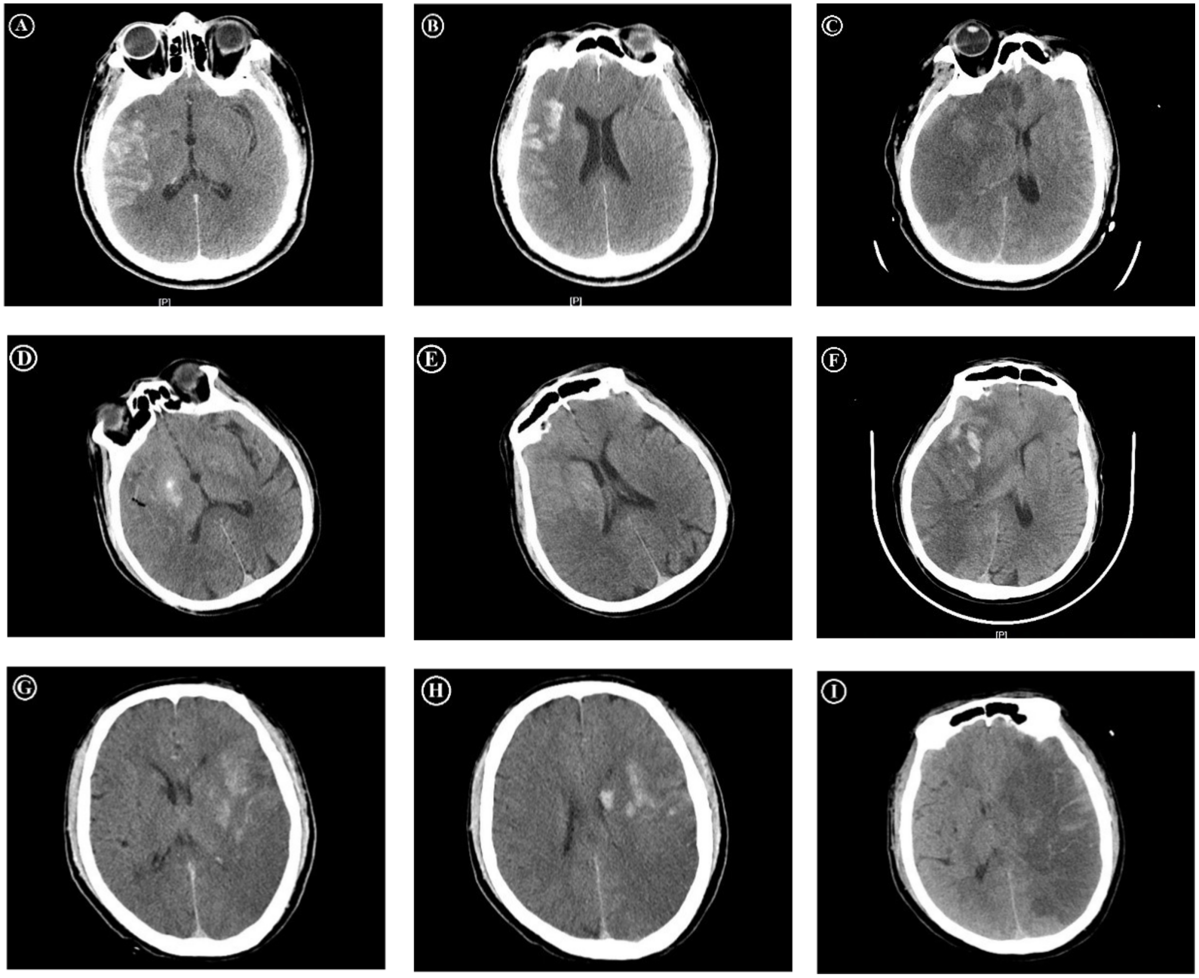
Immediate and follow-up CT images after MT. The first patient, who presented the cortex sign with PCHDS of 7 on CT immediately after MT (A,B), developed MBE on follow-up CT (C). The second patient, who presented the basal ganglia sign with PCHDS of 9 (D,E), eventually developed MBE (F). The third patient, who presented the combined sign with PCHDS of 7 (G,H), eventually developed MBE (I). MT, mechanical thrombectomy; MBE, malignant brain edema; PCHDS, postinterventional cerebral hyperdensities score.
